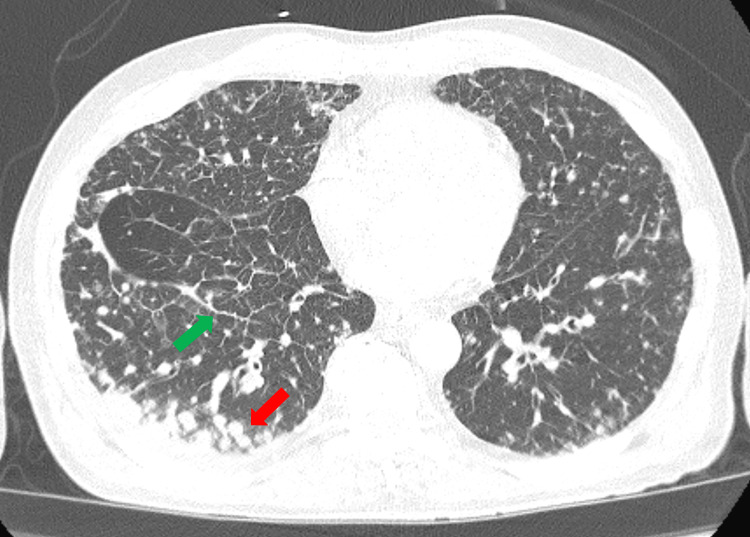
Contrast enhanced computed tomography scan of the chest showing multiple pulmonary nodules (red arrow) within lower lung fields, in addition to intralobular septal thickening (green arrow) in a nodular fashion.
